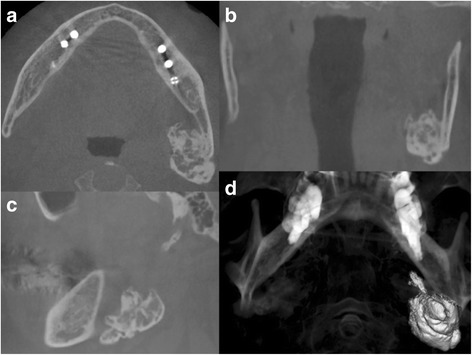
a–c Axial, coronal, and sagittal CBCT scan showing the lesion attached to the inner surface of the mandible. d Three-dimensional cone beam CT image showing an irregular bony mass with an outgrowth at the inferior border of the mandibular angle and extending to the submandibular area. The mass measures 33.3 × 41.0 × 20.2 mm
